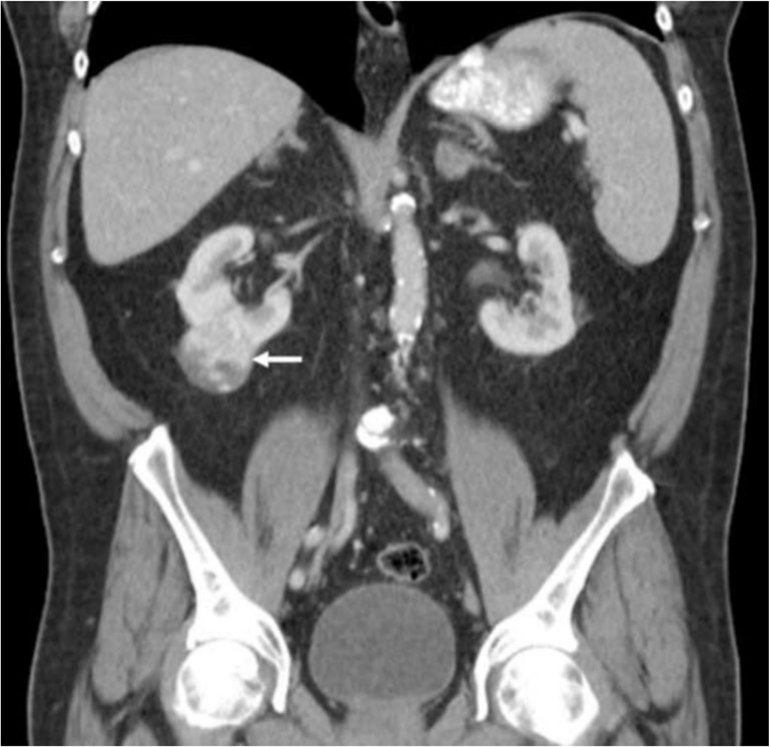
Coronal portal venous CT demonstrating an exophytic heterogeneous renal cell carcinoma arising from the lower pole of the right kidney (*arrow*)
